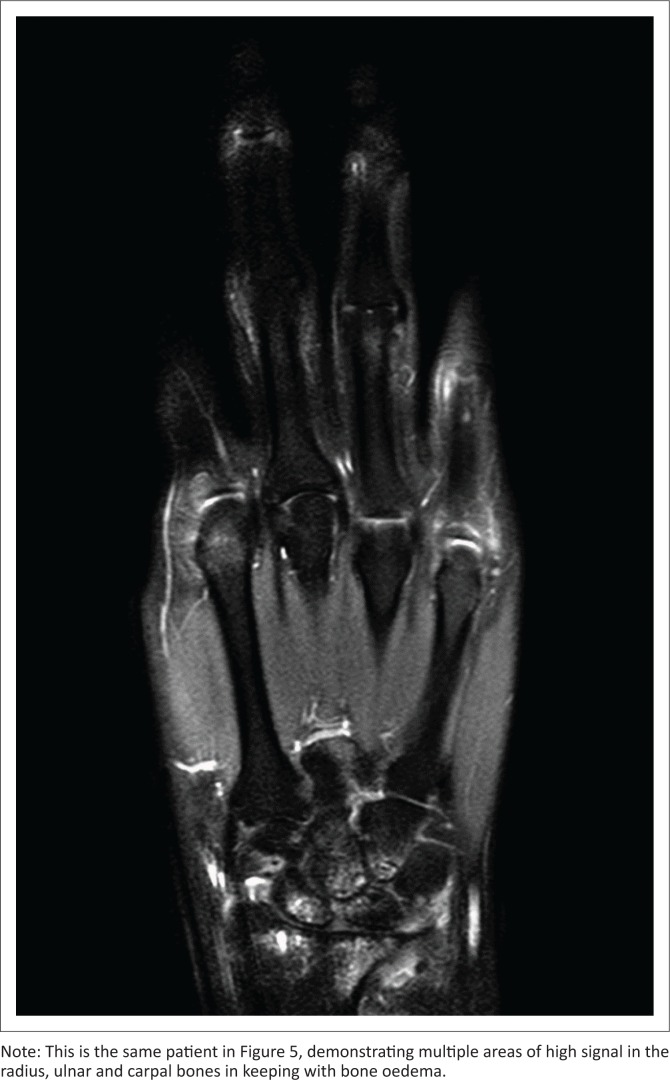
Proton density-weighted fat-saturated coronal magnetic resonance imaging.
